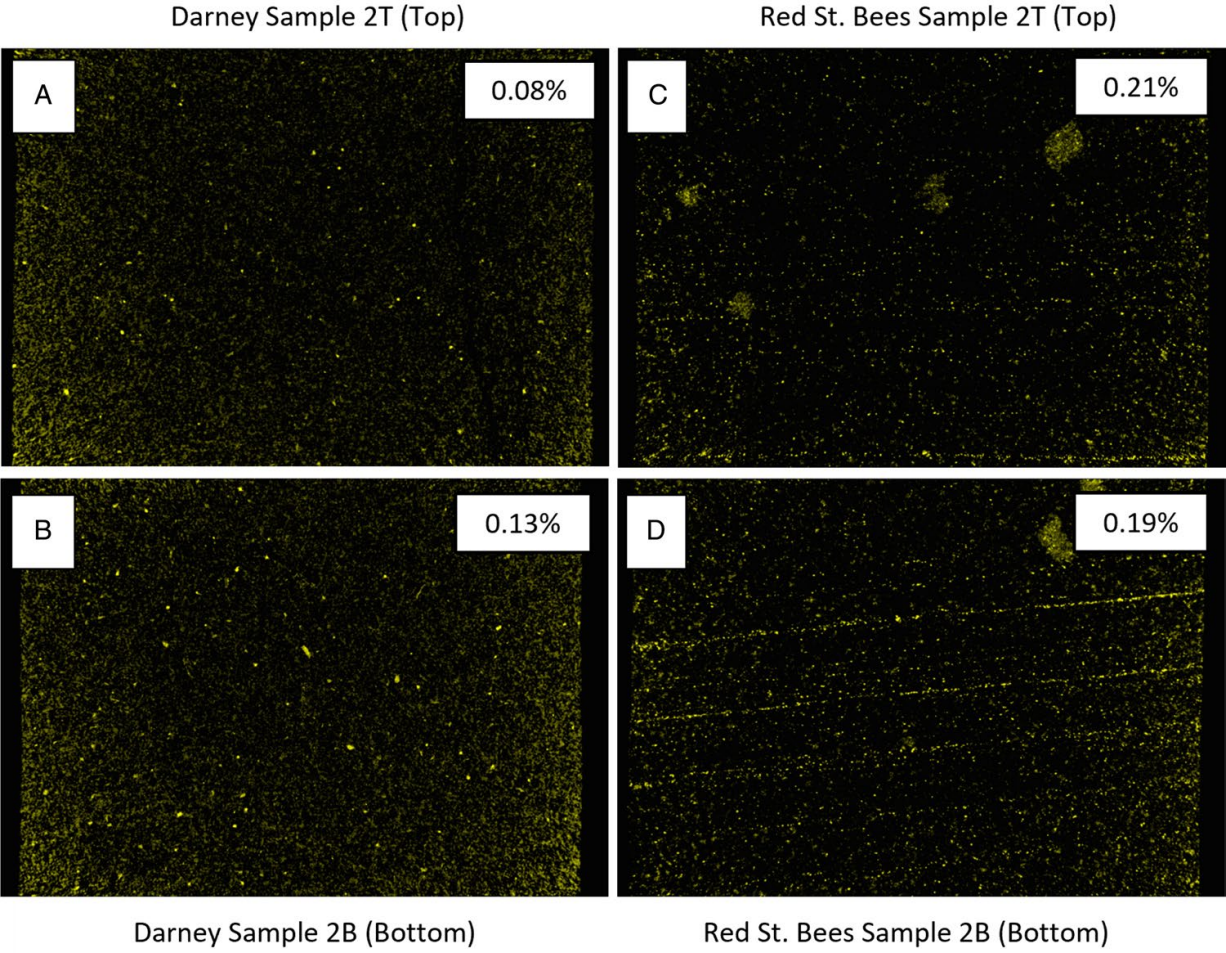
(A) Vertical X-ray reconstruction of high permittivity inclusions within the Darney 2 T sample with a volume content of 0.08% (B) Darney 2B sample with a volume content of 0.13% (C) Red St. Bees 2 T sample with a volume content of 0.21% (D) Red St. Bees 2B sample with a volume content of 0.19%. Each sample is 38 mm in diameter.
